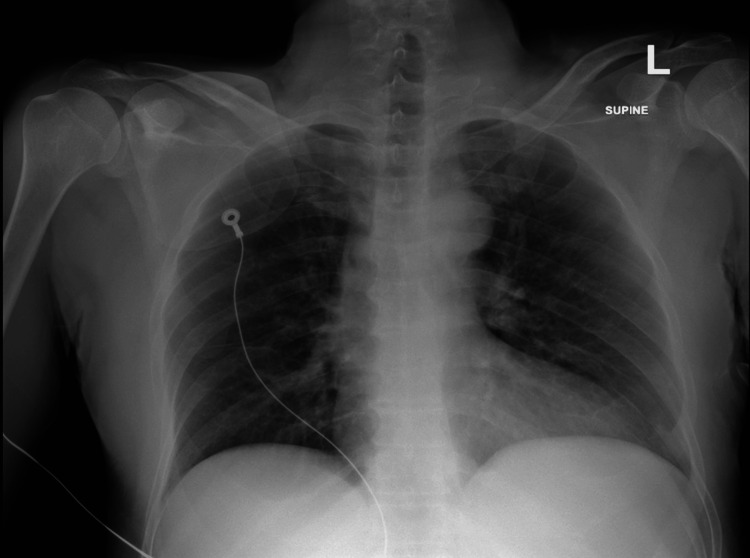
Chest radiograph demonstrating an ill-defined left retrocardiac opacity with associated aortic unfolding.
